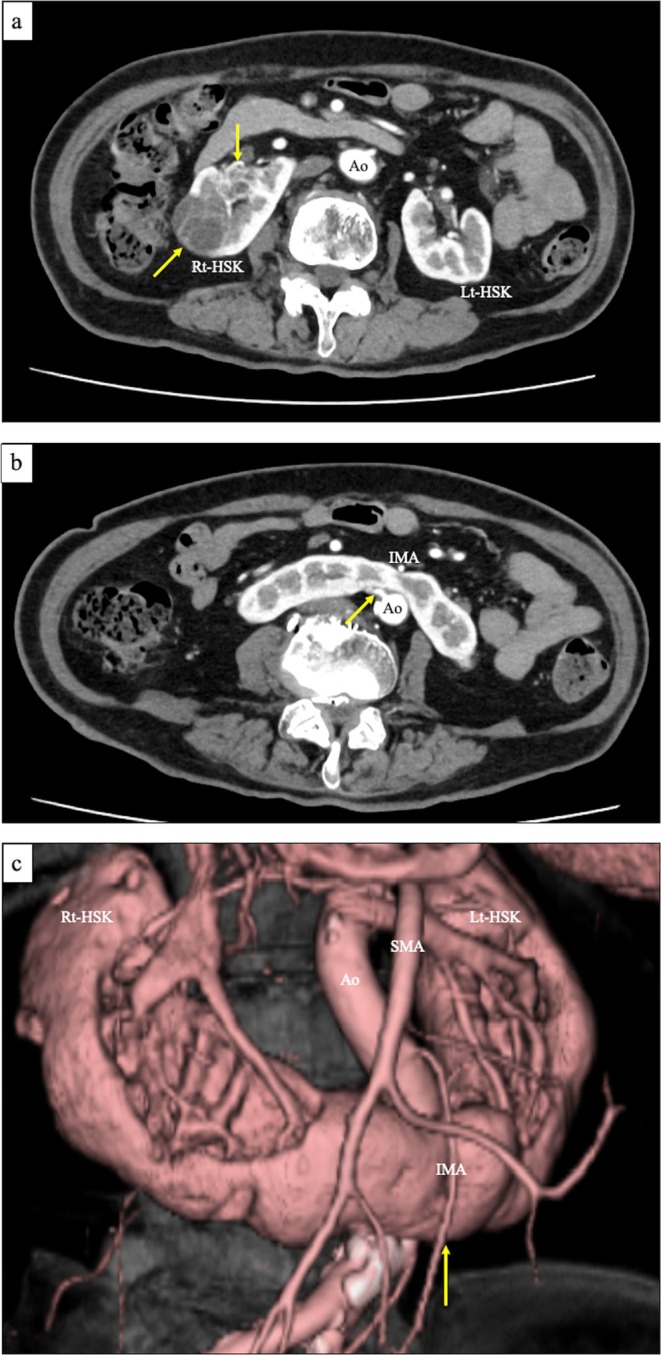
Preoperative contrast‐enhanced computed tomography findings. (a) Two renal tumors located on the right side of the horseshoe kidney (arrows). (b) A renal artery originating from the aorta enters directly into the posterior aspect of the isthmus (arrow). (c) The inferior mesenteric artery crosses the anterior aspect of the isthmus (arrow).
